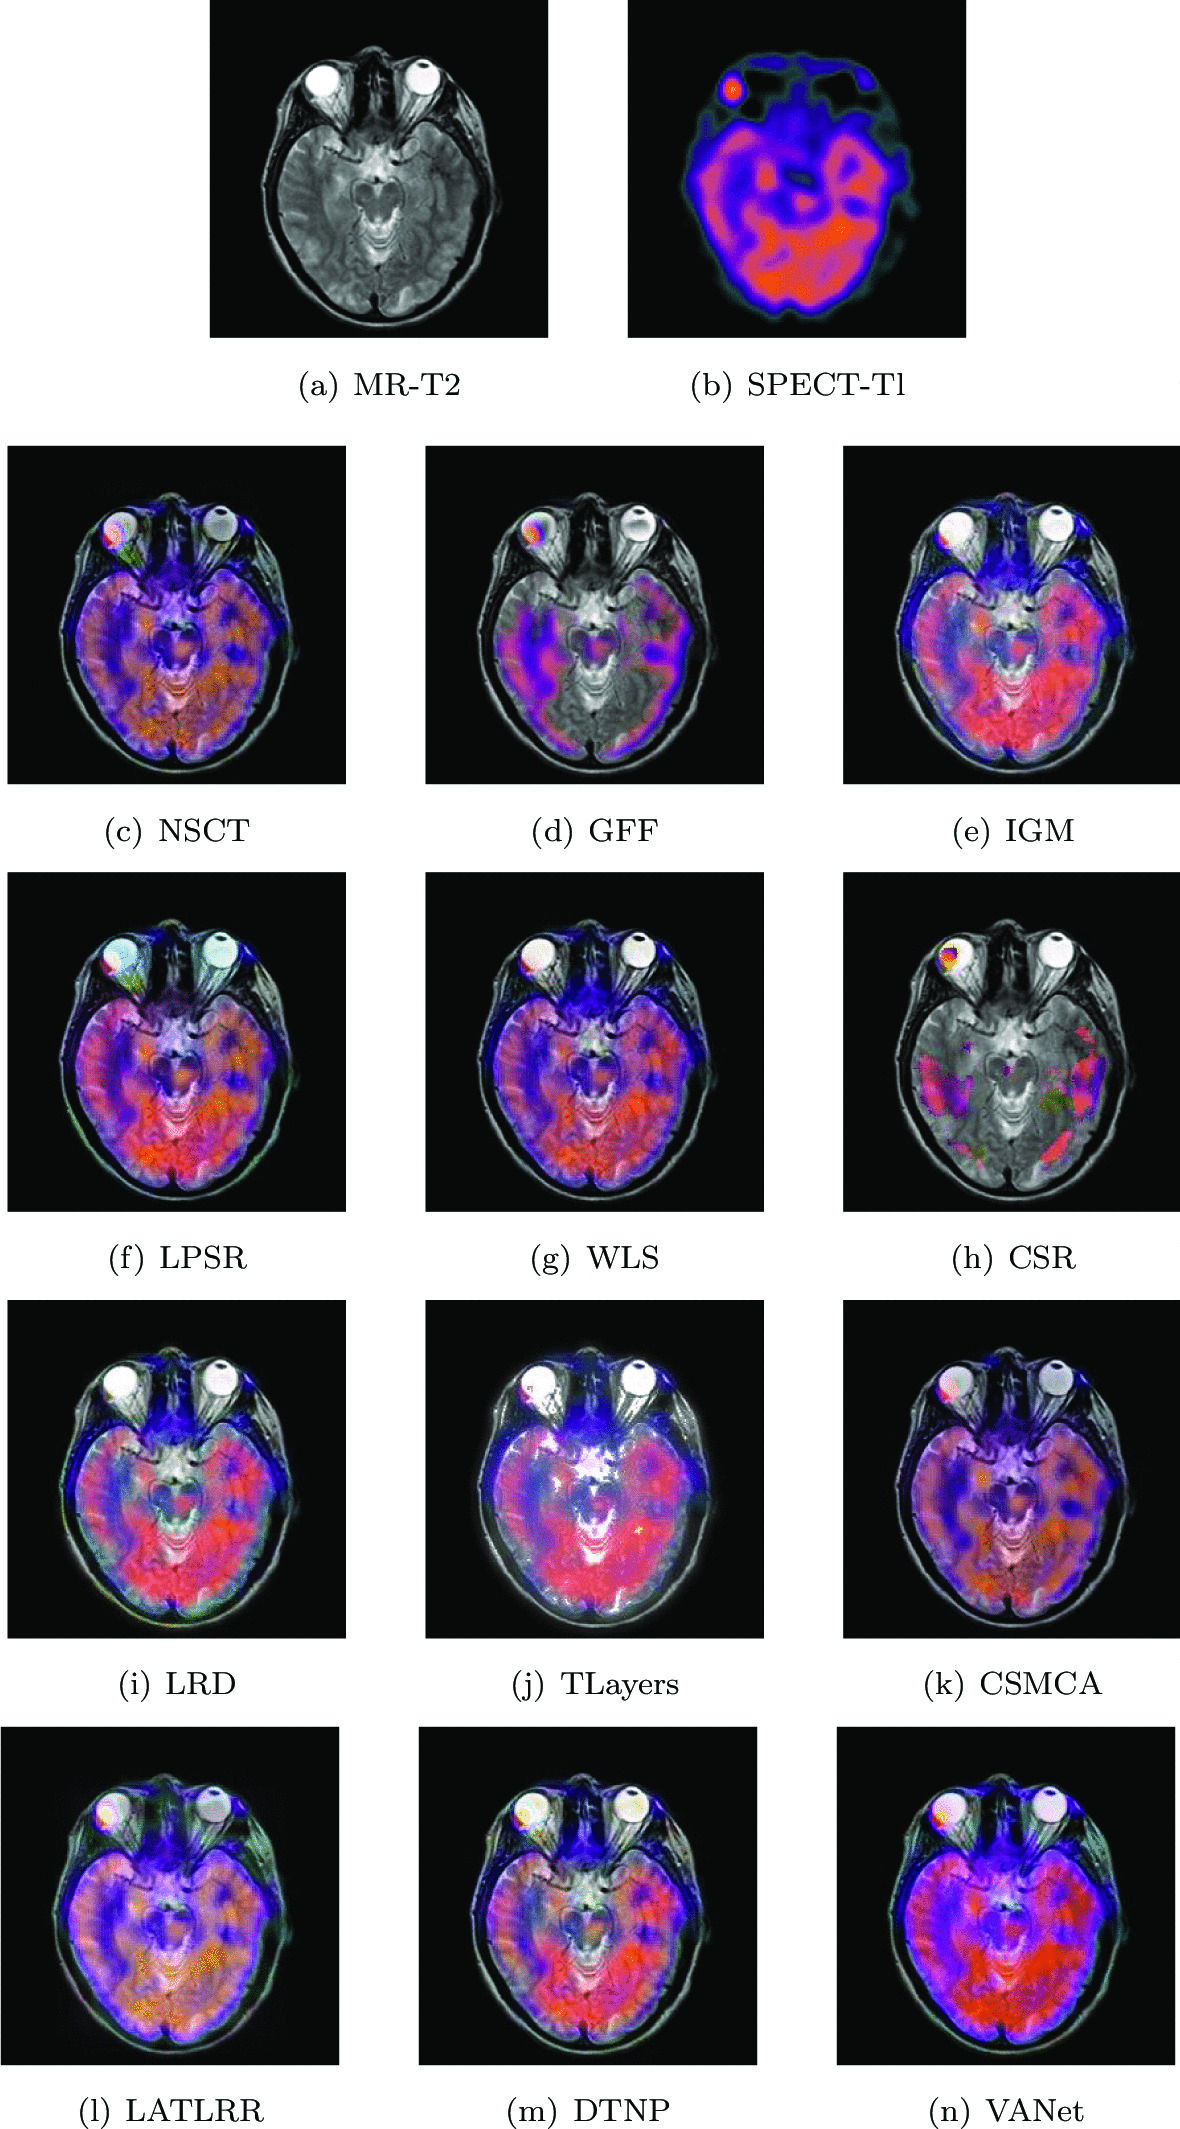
The first set of fused MRI-SPECT images from 9 methods on hypertensive encephalopathy
